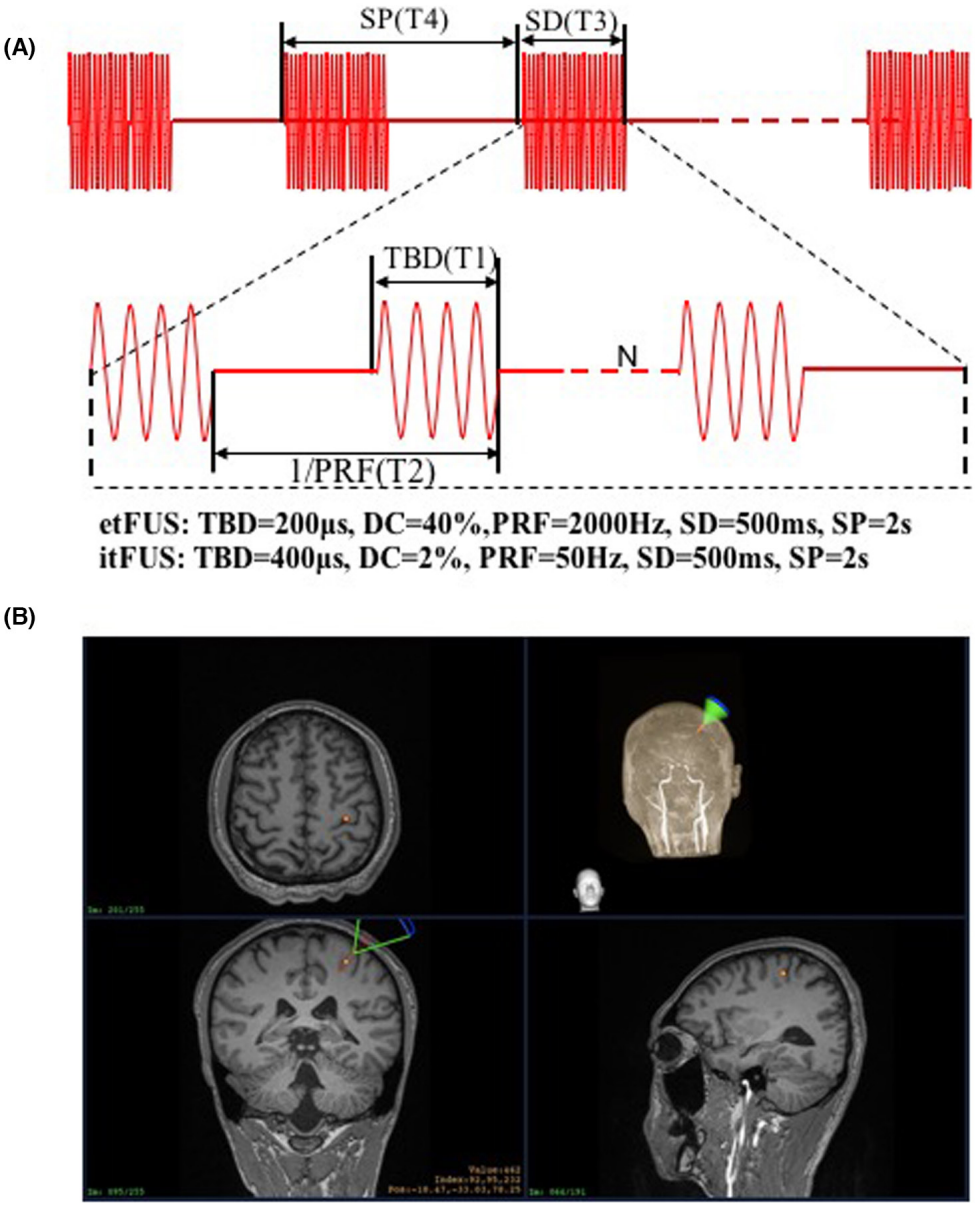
(A) The pulse parameters of tFUS. (B) MRI image guided tFUS focusing over left M1. PRF, pulse repetition frequency; SD, sonication duration; SP, Stimulus Period; TBD, tone‐burst duration.
